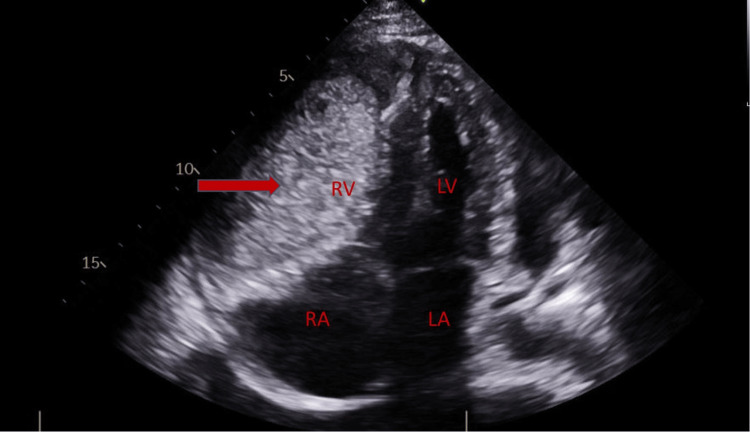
TTE showing an echogenic intrapericardial mass compressing the right ventricle to near obliteration (red arrow) The chambers of the heart are labeled. TTE - transthoracic echocardiogram; RV - right ventricle; LV - left ventricle; RA - right atrium; LA - left atrium
